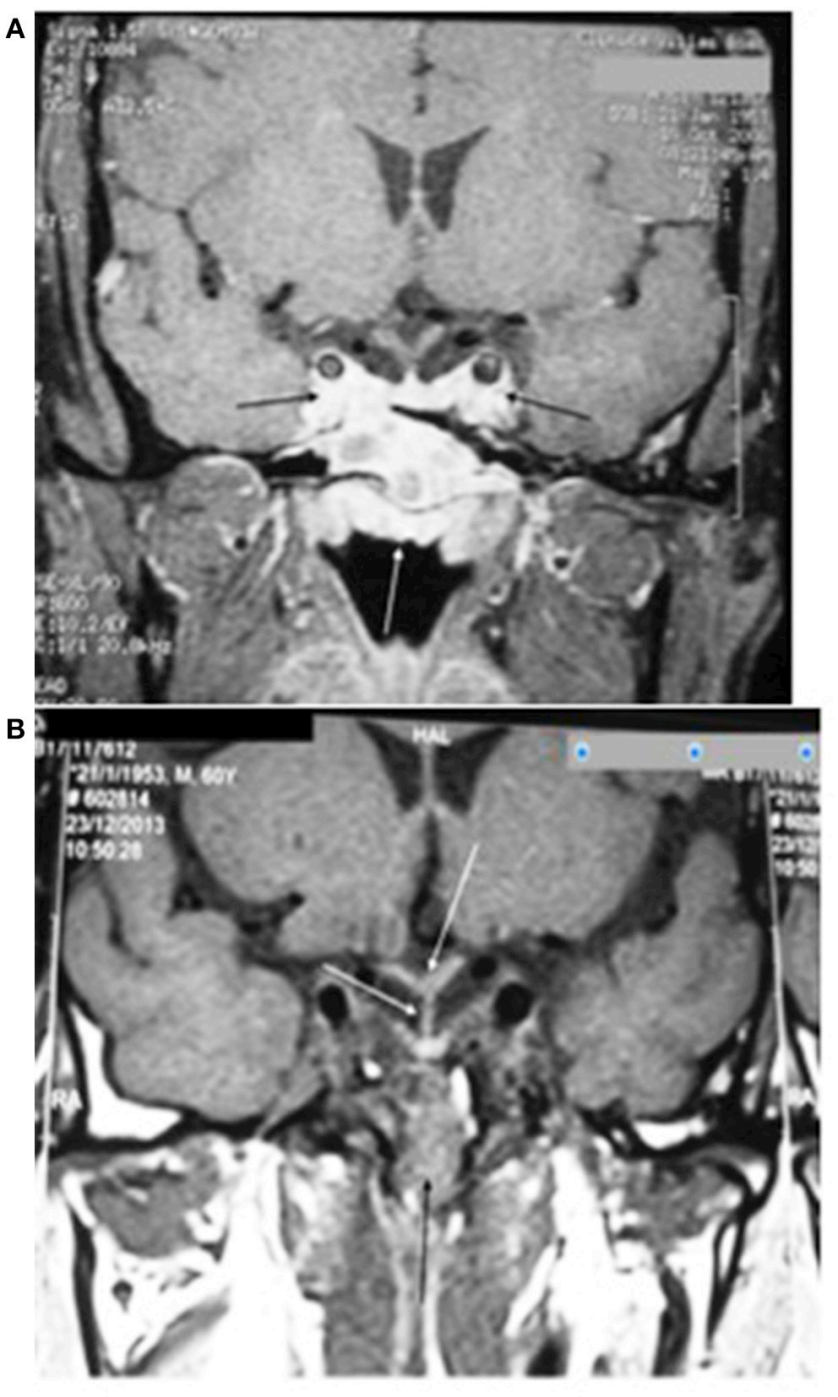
(A) Magnetic resonance imaging of the sella turcica on T1 coronal with heterogeneous contrast after 52 months of treatment with dopamine agonists shows a reduction in the pituitary adenoma with invasion of the cavernous sinuses (black arrows) and total invasion of the sphenoid sinus (white arrow). (B) After 11 years of treatment with dopamine agonists and 5 years after transsphenoidal resection and radiotherapy, the pituitary adenoma is almost totally reduced, but still present in the sphenoid sinus (black arrow); the centered pituitary stalk and pituitary gland and invagination of the optic chiasm (white arrows) can also be seen.
